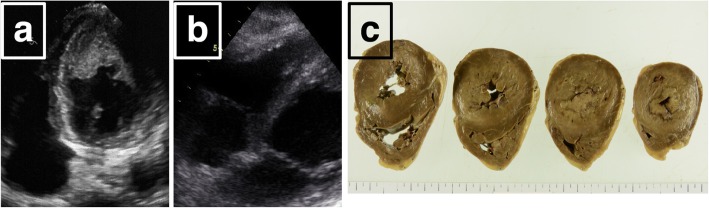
Intramural thrombus in left (a) and right ventricle (b) detected by transthoracic echocardiography. / Formalin-fixation of left ventricle (c). Both ventricular chambers were narrowed by the thrombus. Scale bar: 100µm
